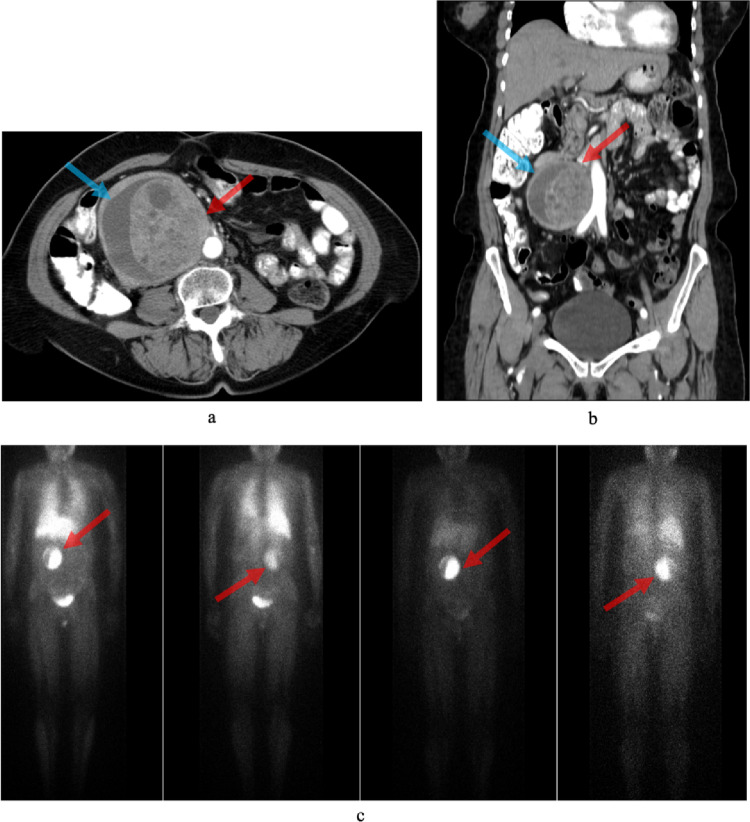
Axial (a) and coronal (b) contrast-enhanced CT scans through the abdomen demonstrate a large predominantly solid right retroperitoneal mass (red arrow) with a smaller crescentic cystic component (blue arrow) at the level of the organ of Zuckerkandl. A subsequent I-123 MIBG scan (c) shows radiotracer uptake within the larger solid component (red arrows). A diagnosis of paraganglioma was confirmed on surgical resection
